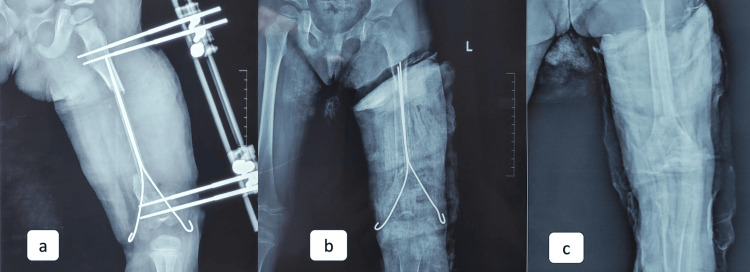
Postoperative radiograph (a) showing the fibular graft held in situ with K-wires and LRS; AP view radiograph at six weeks (b) following removal of LRS; AP view radiograph after eight weeks (c) following removal of K-wires (limb supported by a plaster of Paris slab). AP, anteroposterior; LRS, Limb Reconstruction System.
